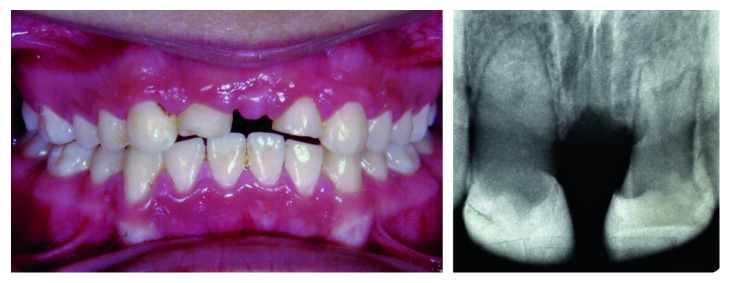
Radiographic and clinical view at the 6-year follow-up.
